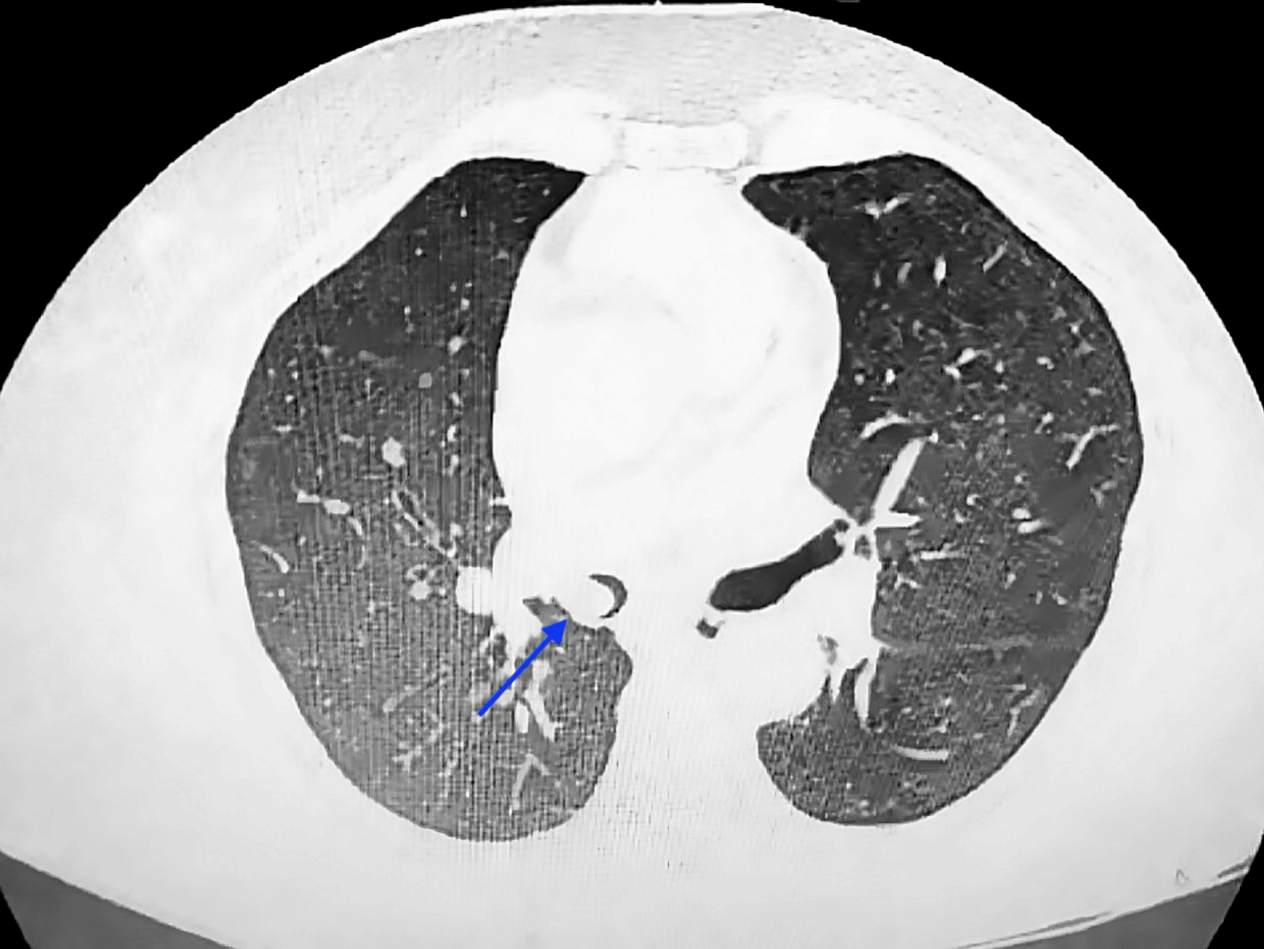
Axial HRCT of the thorax showing an endobronchial lesion in the right main bronchus Lung window HRCT axial view shows a homogeneous fat-density lesion (HU-65) in the right main bronchus (blue arrow), causing partial lumen obstruction. HRCT, High-Resolution Computed Tomography
